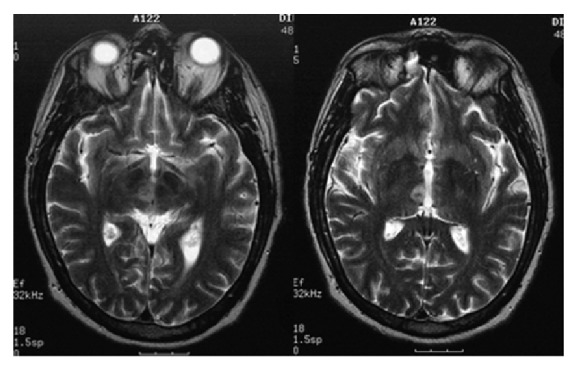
Brain MRI (T2 weighted) of the patient SD showing the acute ischemic lesion confined to the right paramedian thalamus extending to the mesencephalic tegmentum.
